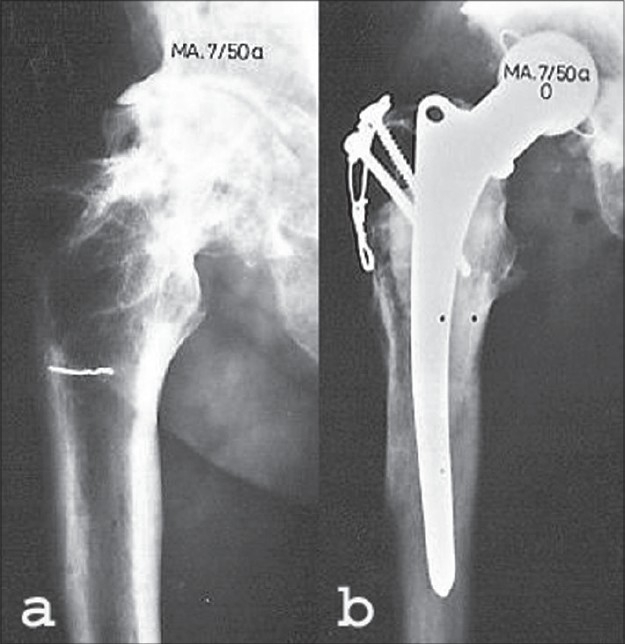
(a) Female 71 years, 16 years after valgization osteotomy; osteoarthritis secondary to avascular necrosis. The sclerotic changes in the femoral head and acetabulum improve the chances for a good longterm result of THR. (b) Postoperative X-ray after cemented THR
